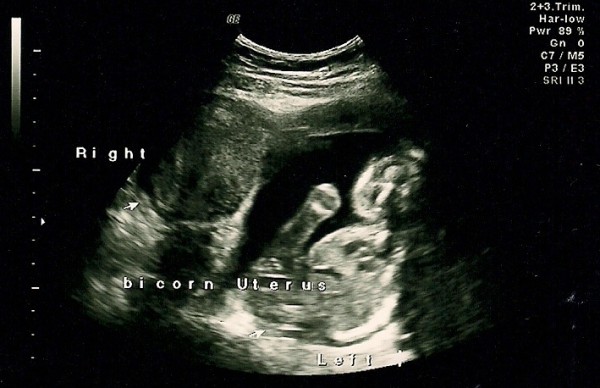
Ultrasonography picture at 19 weeks showing fetus, amniotic fluid and the possibility of a bicornute uterus.
